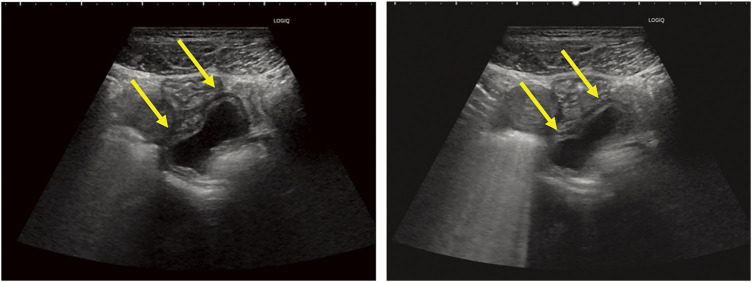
Abdominal ultrasonography showed a cystic lesion with a laminar structure and peristalsis in the upper part of the bladder.
